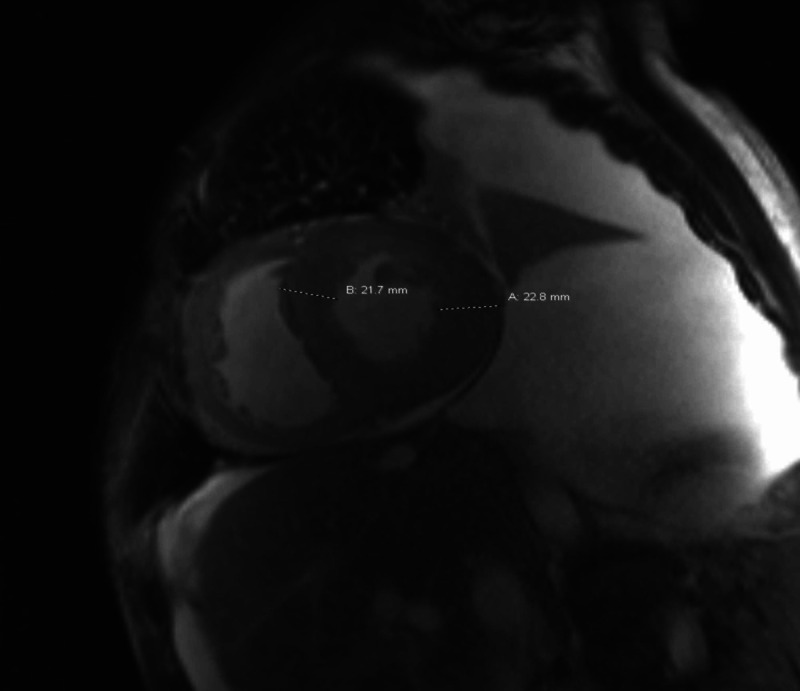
Cardiac MRI Concentric left ventricular myocardial thickening measuring over 20 mm, up to approximately 23 mm in the lateral wall (normal mid cavity thickness is 5-9 mm).
